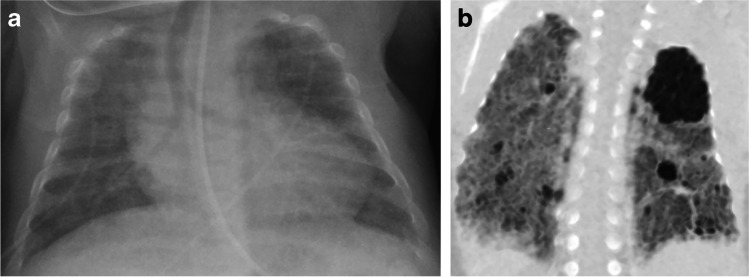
Lung findings of bronchopulmonary dysplasia on radiography and CT. a Anteroposterior (AP) chest radiograph of a premature infant girl born at 23 weeks of gestation, now post-menstrual age 38 weeks (15 weeks old). Portable chest radiograph shows diffuse increased density throughout the lungs but no evidence of air-trapping or cystic changes. b Coronal oblique minimum-intensity projection reconstructed CT image demonstrates hyperlucent lung in the left upper lobe and small cysts throughout the lungs. CT also suggested tracheomalacia was present (not shown)
